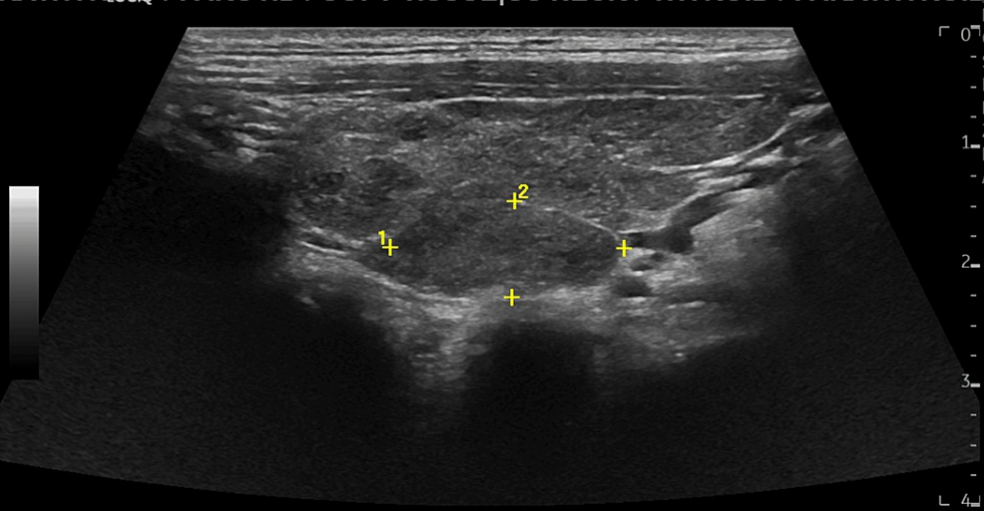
Right thyroid nodule A 2.2 x 1.0 cm hypoechoic homogenous nodule with well-defined borders was identified on ultrasound examination of the neck.
